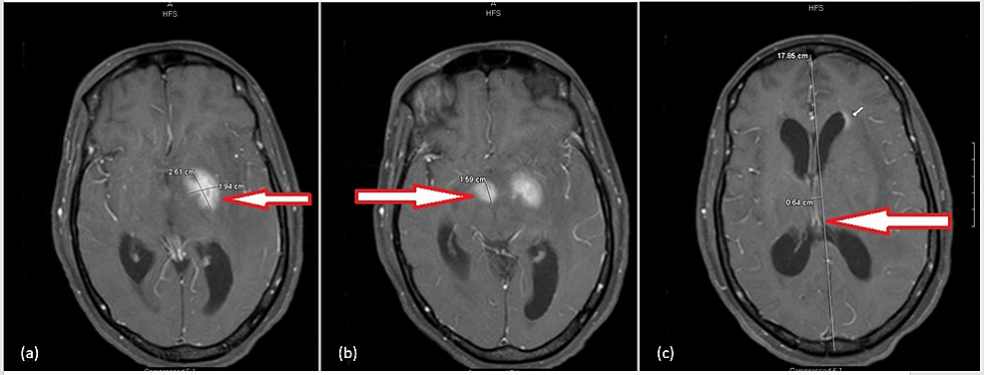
Contrast-enhanced MRI images for Case 1 All sagittal views demonstrating enhancing component of masses: (a) measuring 2.6 x 1.9 x 2.1 cm in the left globus pallidus; (b) measuring 1.7 x 1.6 by 1.0 cm in the hypothalamus; (c) with a 6 mm right to left midline shift
